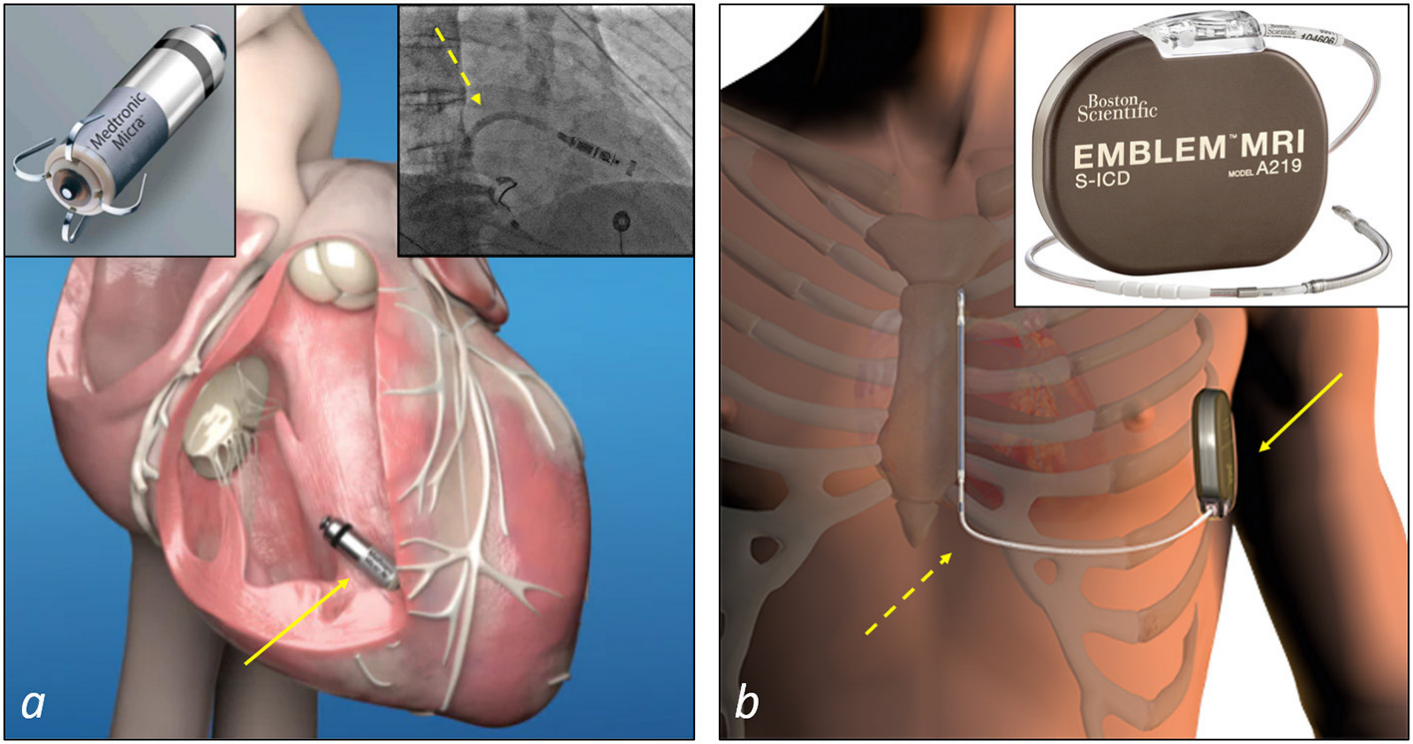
Examples of non-transvenous CIEDs systems to deliver cardiac pacing or defibrillation therapy. (a) A leadless PM (Micra™ Medtronic, top-left corner) and the site of implant at the apical-septal level of the right ventricle (arrow). The insert at the top right corner shows a step of a Micra™ implantation: the catheter delivery system (dashed arrow) is advanced into the right ventricle to deliver the device. (b) A subcutaneous ICD (Emblem™ MRI Model A219, Boston Scientific, top-right corner) and the site of implant of the device (solid arrow) and the lead (dashed arrow). The device is implanted in the left axillary region in an intermuscular pocket created between the serratus anterior and the latissimus dorsi muscles, and connected to the lead implanted in the subcutaneous tissue of the parasternal region of the chest.
